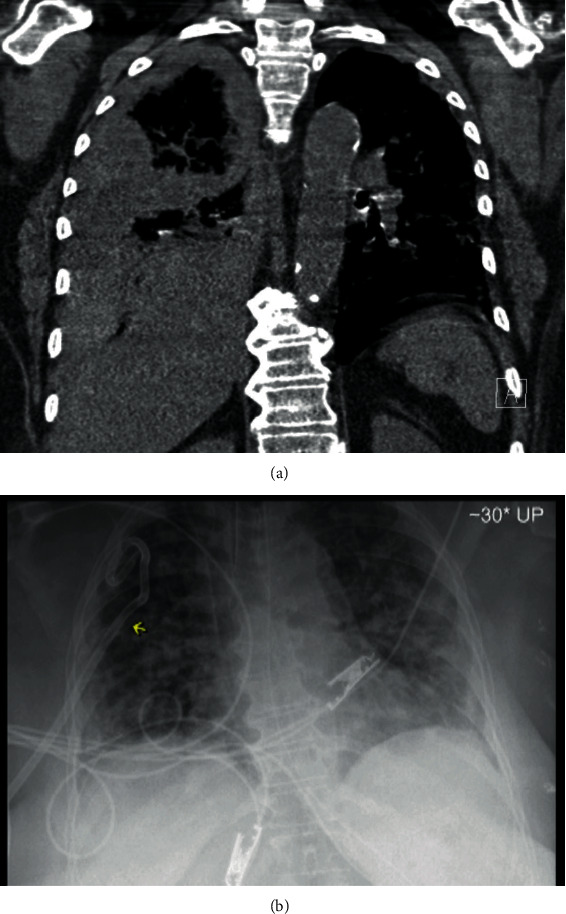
(a) Chest CT showing large right-sided hemothorax as a complication of therapeutic anticoagulation for pulmonary embolism. (b) CXR showing reexpanded lung on the right after placement of the chest tube.
